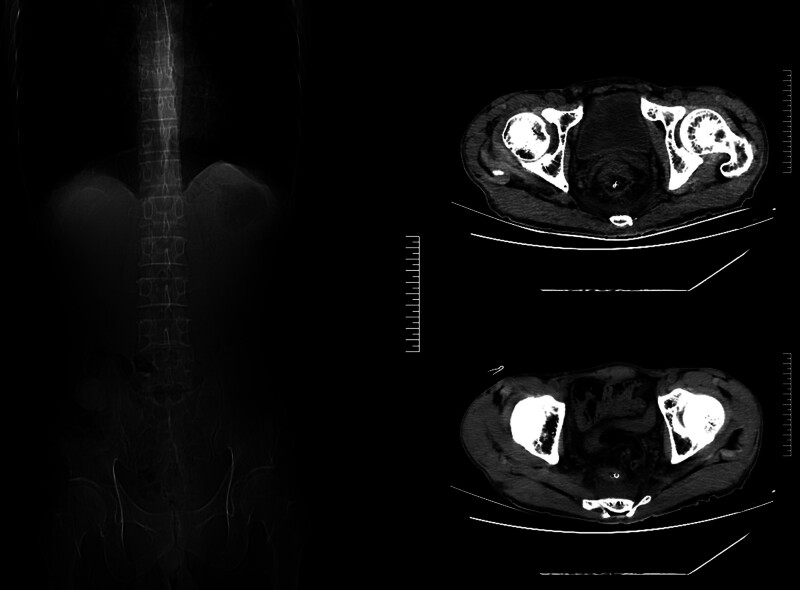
Computed tomography (CT) on January 26, 2024.
